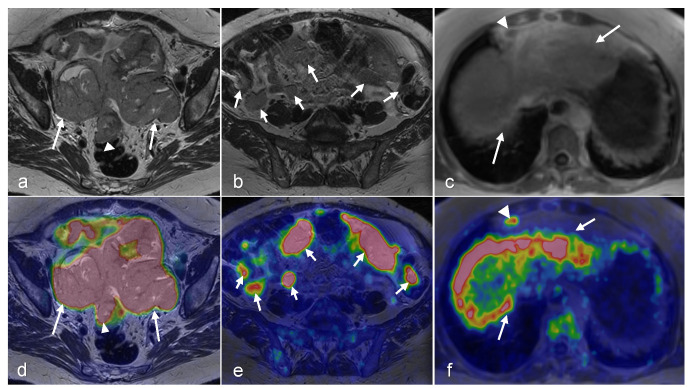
A 63-year-old woman with peritoneal carcinomatosis secondary to bilateral high-grade serous carcinoma with a PCI score of 39 with invasion of the rectal wall (arrowhead in (a,d)). On axial T2-weighted images, massive infiltration of the greater omentum is visible (arrows in (a,b)) with high FDG uptake (arrows in (d,e)) on axial-fused PET/MRI images. Both axial T1-weighted in-phase (c) and axial-fused PET/MRI (f) demonstrate multiple peritoneal implants spread across the surface of the liver, carcinomatosis implants on the diaphragm (arrows in (c,f)), and one supradiaphragmatic lymph node metastasis (arrowhead in (c,f)). (Courtesy of Elisabeth Hedlund, MD, Håkan Ahlström, MD, and Björg Jónsdóttir, MD, PhD, Uppsala University, Uppsala, Sweden).
